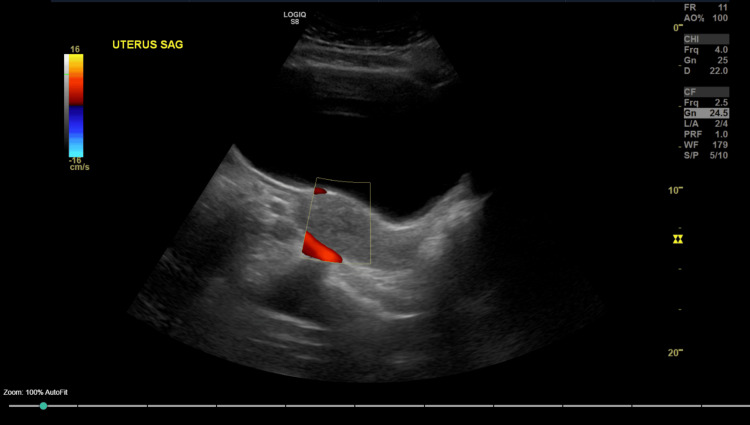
Sagittal ultrasound of the uterus demonstrating no evidence of retained products of conception
